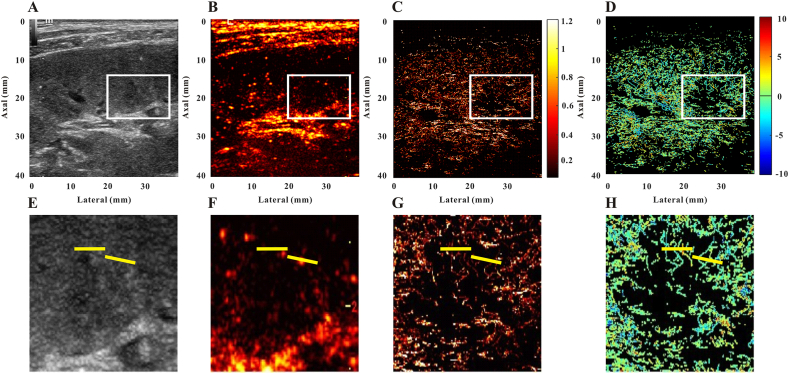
Representative images of a normal allograft patient. B-mode image (A), contrast-enhanced ultrasound image (B), super-resolution image (C), super-resolved velocity image (D), and the zoomed-in regions indicated as the red box of the B-mode image (E), contrast-enhanced ultrasound image (F), super-resolution image (G), super-resolved velocity image (H). Yellow lines highlight the resolution improvement among the images.
